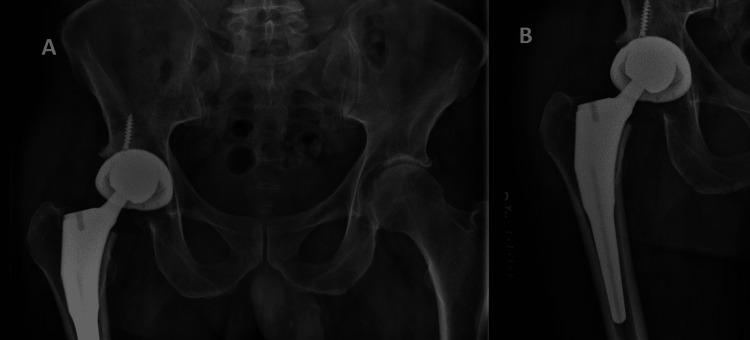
(A) AP radiograph of pelvis and (B) hip showing PLD AP: antero posterior, PLD: polyethylene liner dissociation
